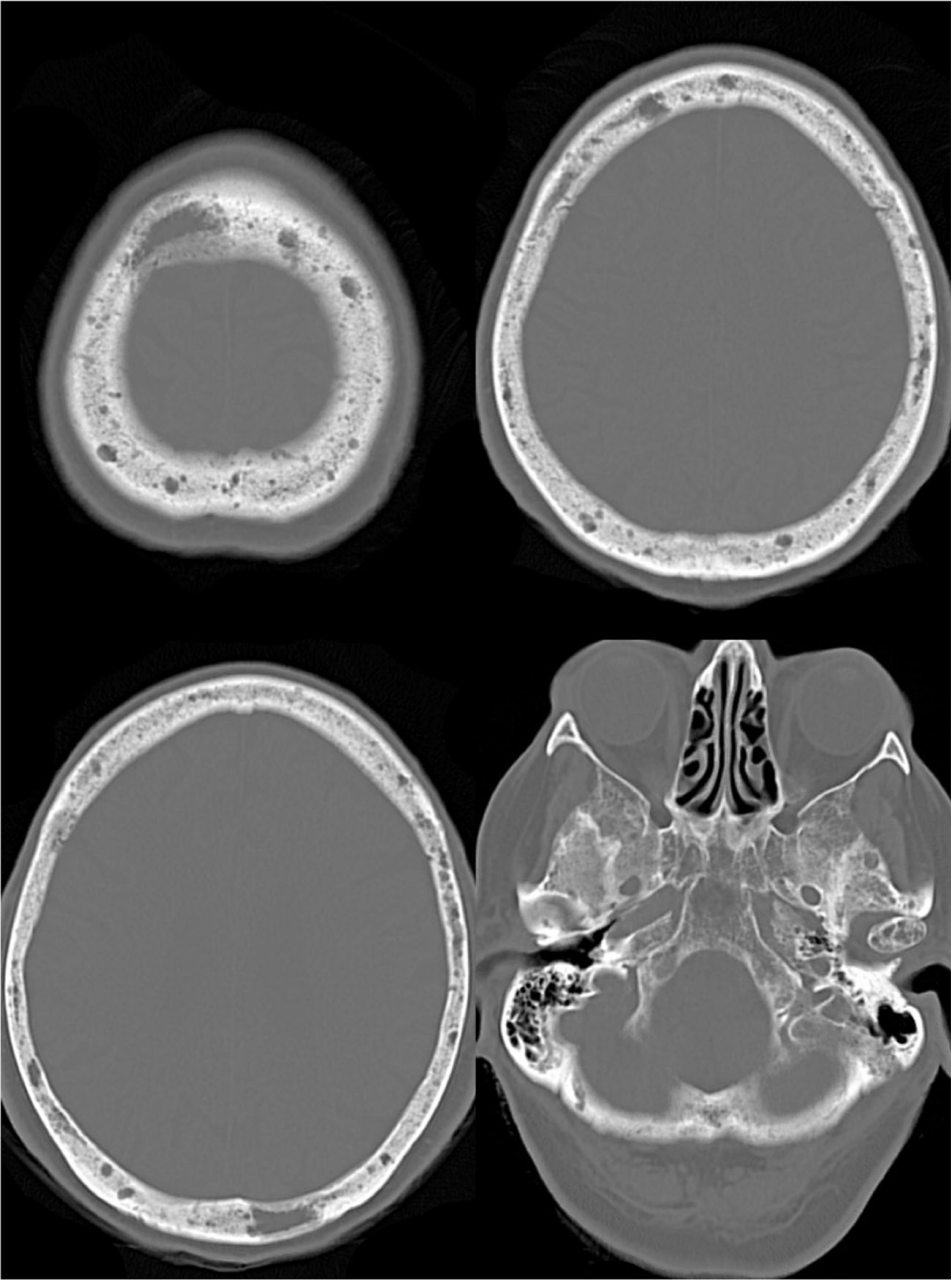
Multiple myeloma (MM). Axial CT images with bone window showing multiple osteolytic lesions varying in shape and size, involving the skull vault and base
